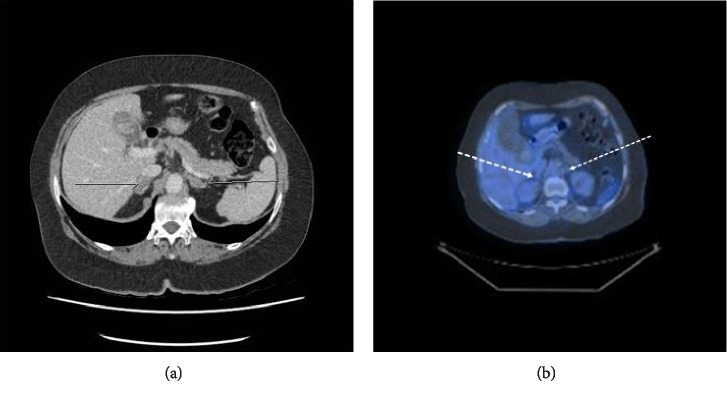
(a) Axial noncontrast CT image following six cycles of primary chemotherapy demonstrating good response with both adrenal metastases decreased in size (white arrows). (b) The adrenal metastases were no longer PET-avid.
